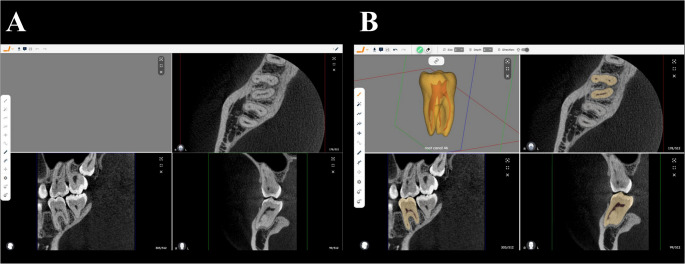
Display of the artificial intelligence (AI)-driven cloud-based platform (creator.relu.eu; Relu, Leuven, Belgium) used according to the imaging assessment approaches: (A) conventional CBCT assessment and (B) CBCT assessment aided by a three-dimensional AI-generated model. CBCT, cone beam computed tomography
